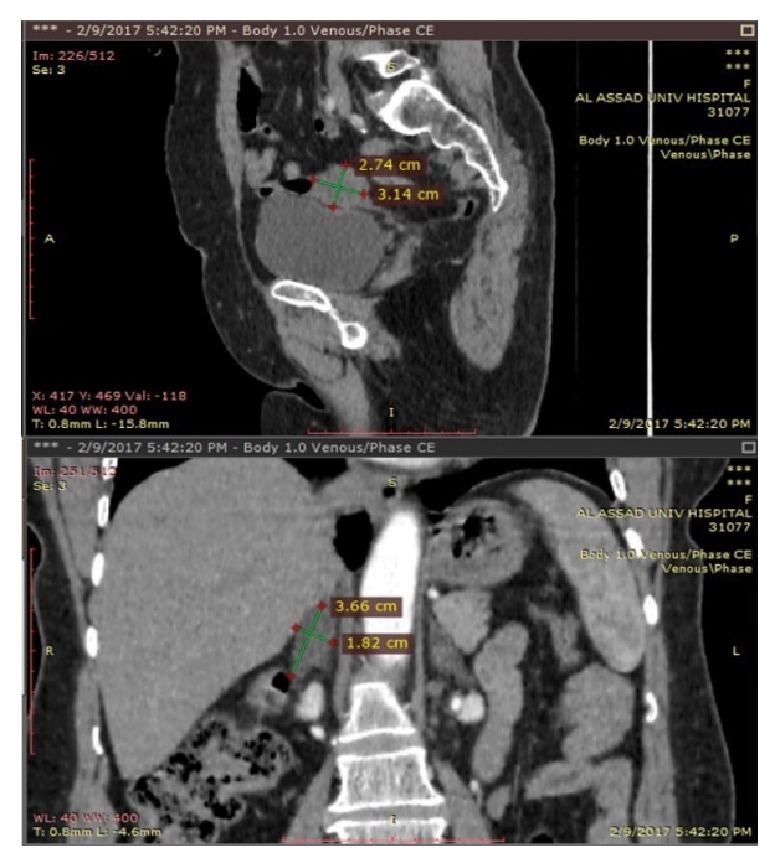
On the top, the figure shows sagittal CT section of the left ovary measures 2.74 cm by 3.14 cm. On the bottom, it shows coronal section adrenals with the largest nodule in right adrenal gland measures 3.66 cm by 1.82 cm with a left adrenal nodule.
